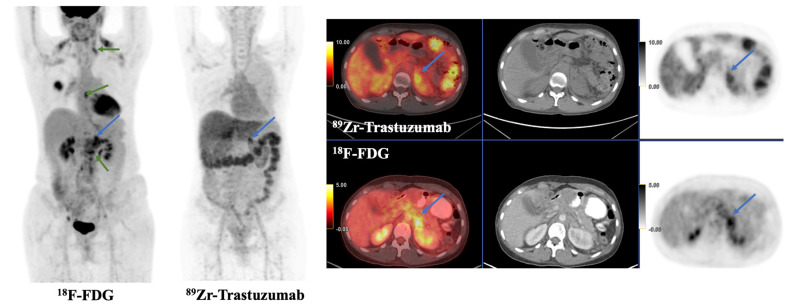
This is a 43-year-old female with left breast invasive ductal carcinoma (ER+, PR+, and HER2−) and metastases to the left supraclavicular, thoracic, and retroperitoneal nodes and left adrenal gland. She was on everolimus and exemestane at the time of ^89^Zr-trastuzumab PET/CT. Tracer avidity was visualized in a known left adrenal metastasis (blue arrow), with SUVmax 9.2. Additional FDG-avid left supraclavicular, thoracic, and retroperitoneal nodes were not tracer avid on ^89^Zr-trastuzumab PET/CT (green arrows), suggesting lack of HER2 expression and HER2 intertumoral heterogeneity of metastatic lesions.
